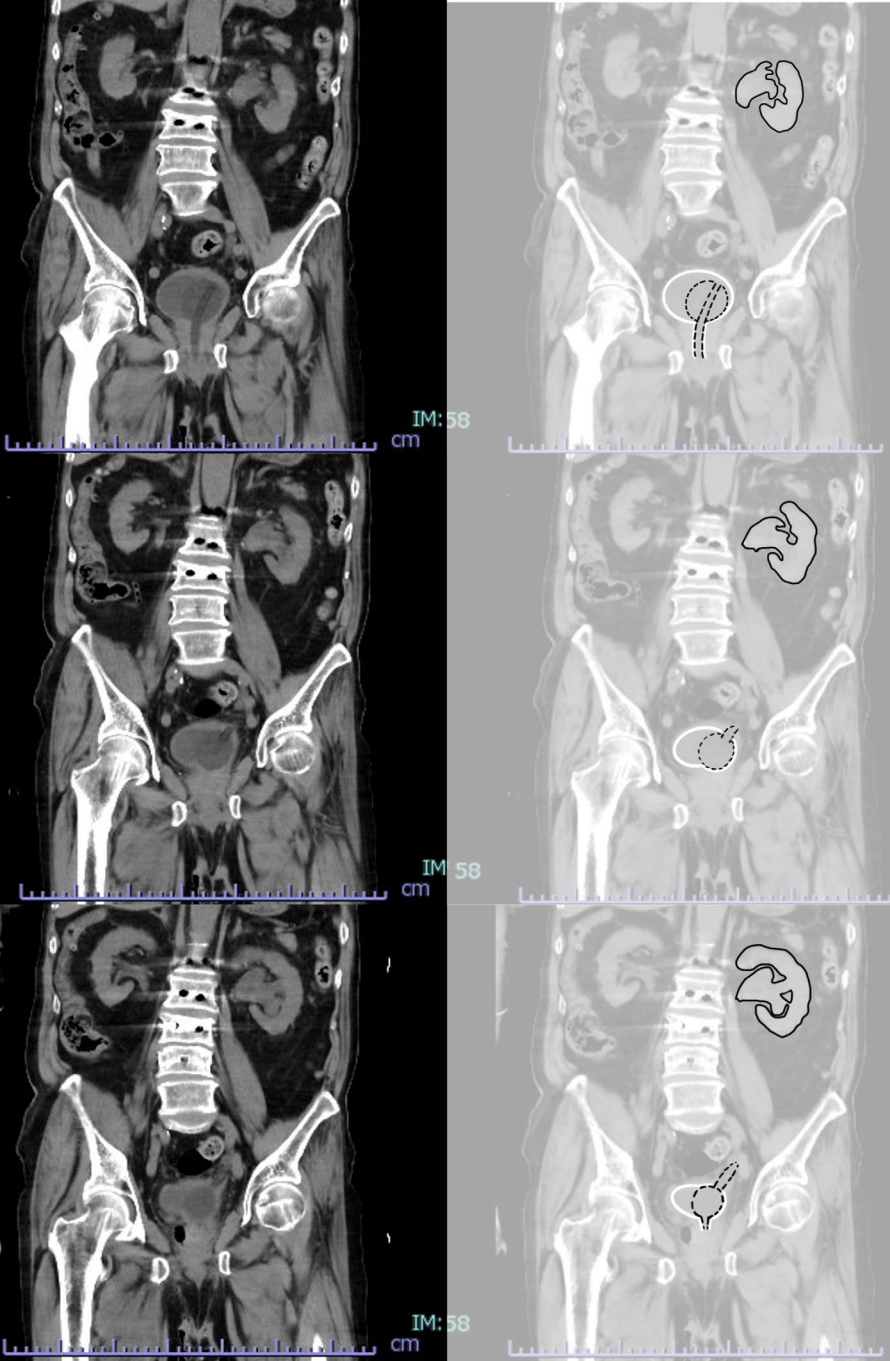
CT (coronal section, at the first stray of the urinary catheter). Black line: left kidney, renal pelvis. Black dotted line: urethral catheter. White line: bladder, urethra. The tip of the urethral catheter strayed into the left ureter, causing left hydronephrosis (SFU grade 3).
